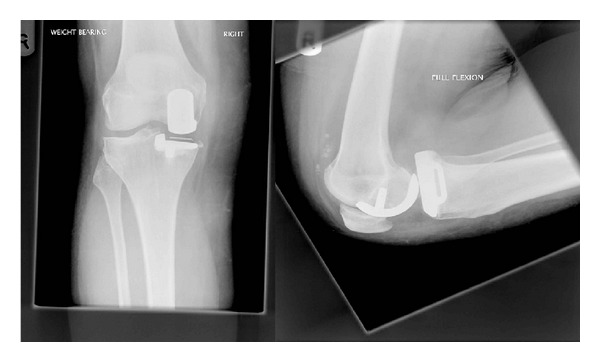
AP view and lateral view radiographs of the knee prior to replacing the mobile bearing.
